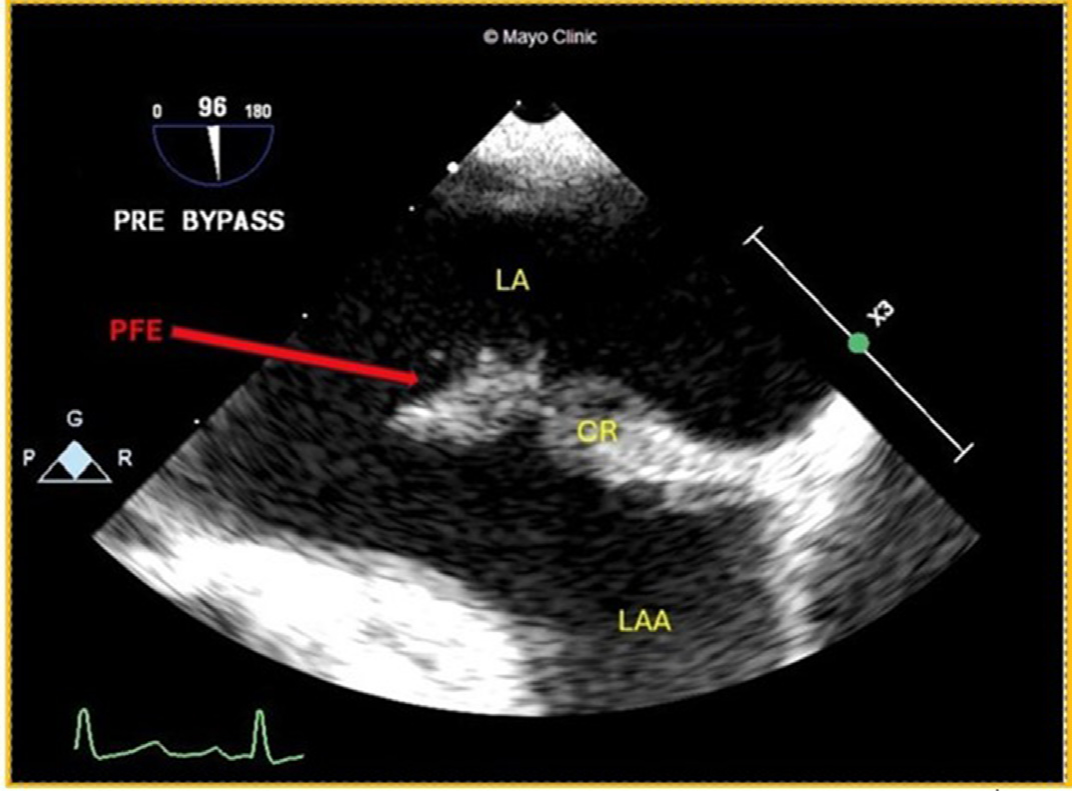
Transesophageal Echo TEE, 2-D mode-focus on LAA, showing the PFE attached to the PVR by a stalk. Abbreviations as in Figures 1 and 2.
